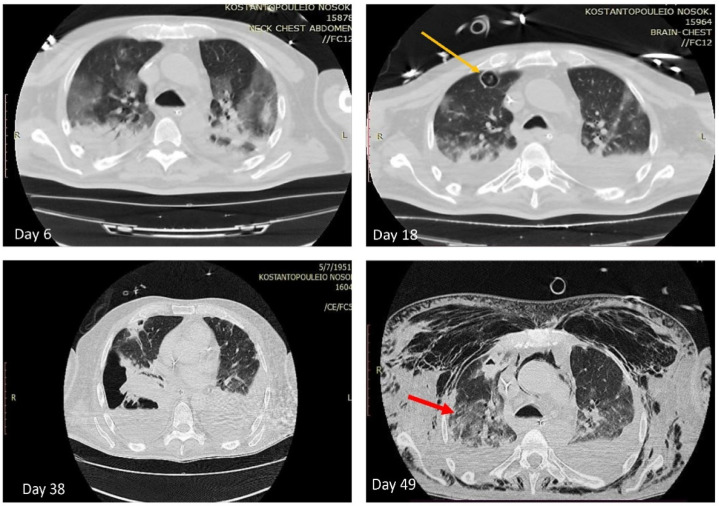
Chest computed tomography (CT) showing multi-lobar peripheral ground-glass opacities and consolidations (day 6); the yellow arrow depicts one cavitary lesion (day 18); the red arrow depicts the bronchopleural fistula (day 49).
